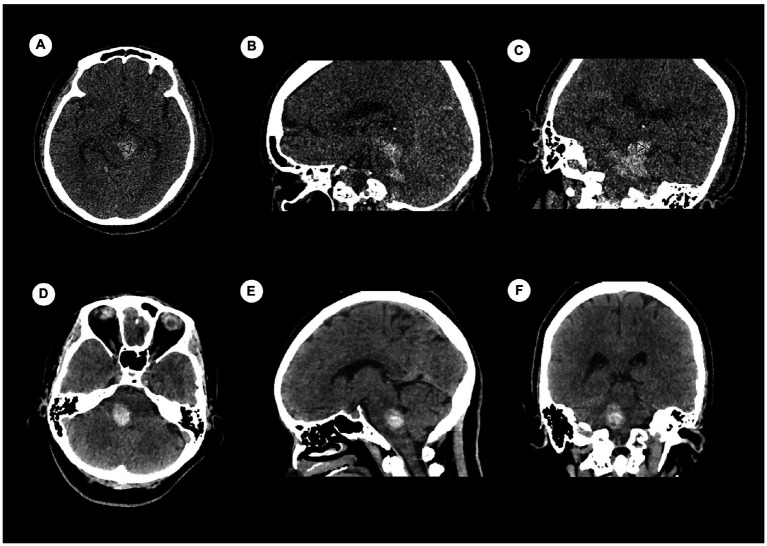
Examples of hemorrhage involving the midbrain (upper) and not involving the midbrain (lower) detected by craniofacial computer tomography (CT) scans. (A) Transverse view: Hematoma was present only in midbrain (▷). (B) Coronary view: Hematomas were present in midbrain (▷) and pons. (C) Sagittal view: Hematomas were present in midbrain (▷) and pons. (D) Transverse view: Hematoma was present only in pons. (E) Coronary view: Hematoma was present only in pons. (F) Sagittal view: Hematoma was present only in pons.
